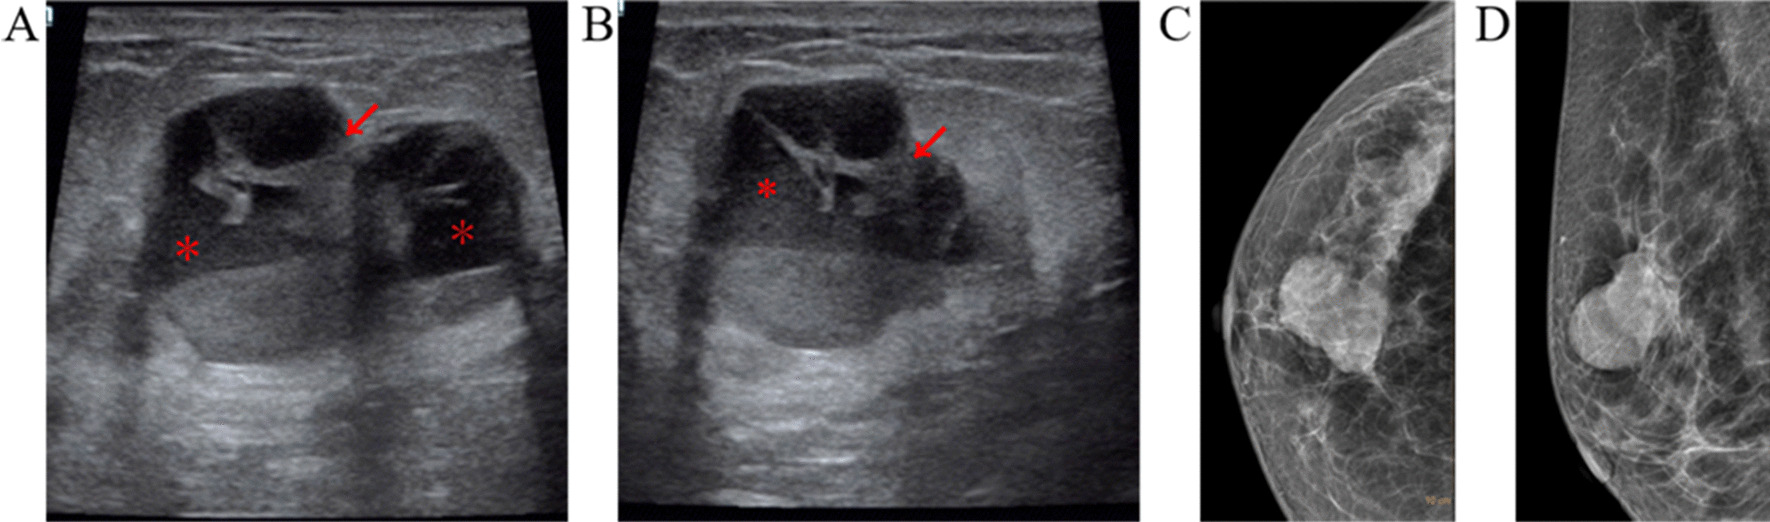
a, b Ultrasonogram revealed no echogenic (red *) and well circumscribed mass with solid striped septum (red ↙), weak echogenic deposition in the dorsal side. c, d On the CC and MLO mammograms showed lobulated mass without calcification
